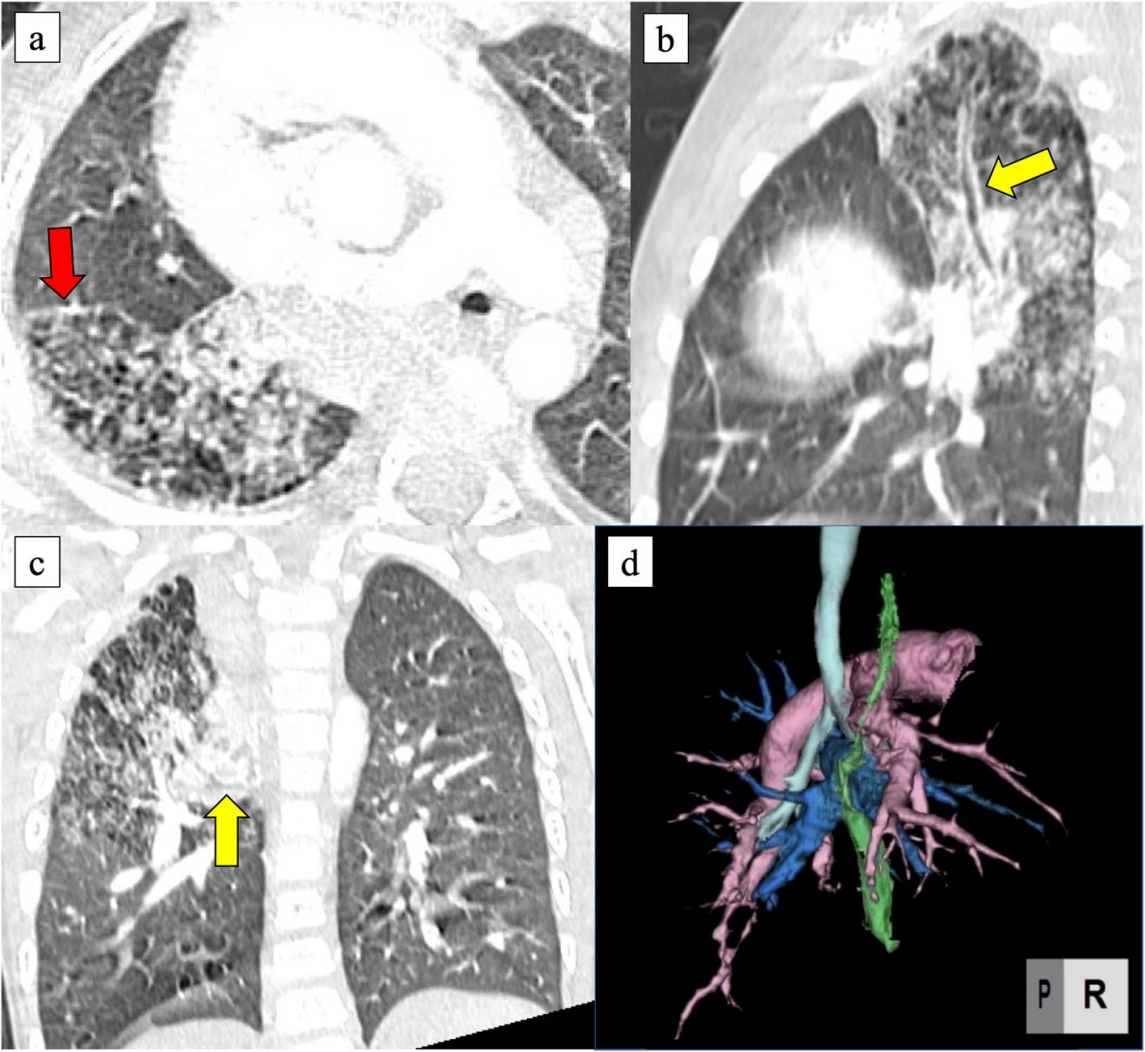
CT scan at 24 months of age. It revealed the aerated RUL with consolidation and esophageal bronchus (yellow arrow). The margin of the affected lung was seen (red arrow). a Axial, b coronal, and c sagittal sections. d 3-dimensional image. Her pulmonary artery and pulmonary vein in the right lung were of normal structure
